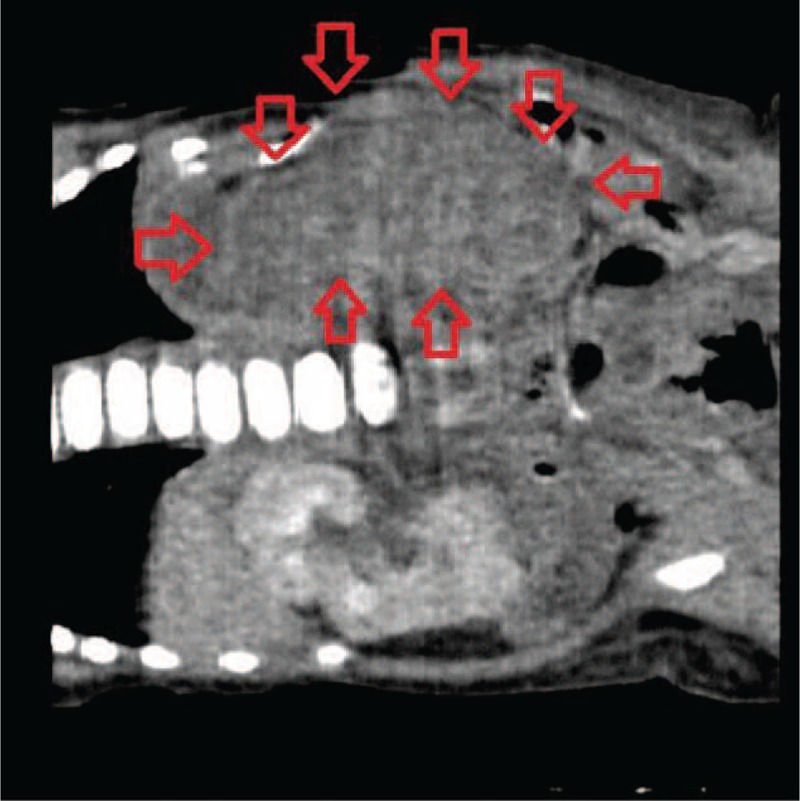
Upper abdominal CT scan after intravenous contrast medium administration. An enlarged left kidney with lack of contrast enhancement was observed.
